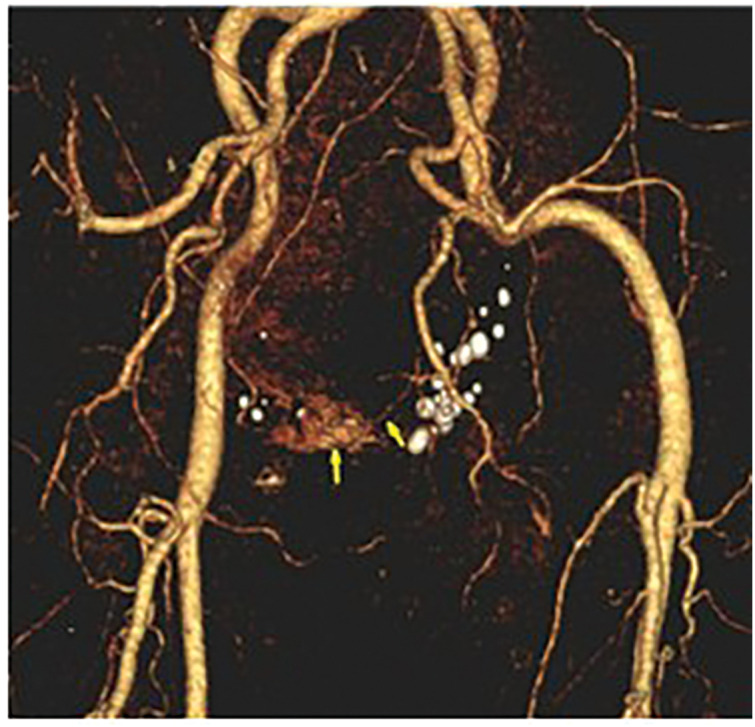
3D-CTA of the pelvic AVM. 3D-CTA demonstrating the nidus of the pelvic AVM (yellow arrows). The right side of the image corresponds to the patient’s right side, viewed from a posterior perspective. AVM, arteriovenous malformation; CTA, CT angiography
